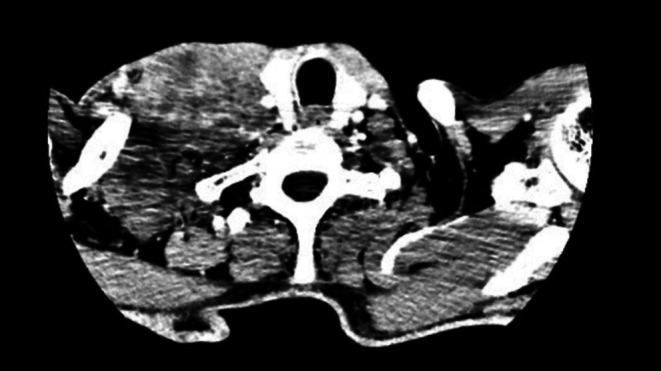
Contrast‐enhanced CT scan of the neck showing a large, ill‐defined lobulated mass in the right supraclavicular fossa and lower neck, abutting the carotid sheath with loss of intervening fat planes and partial encasement of the right subclavian vessels; no adjacent bone erosion is noted.
